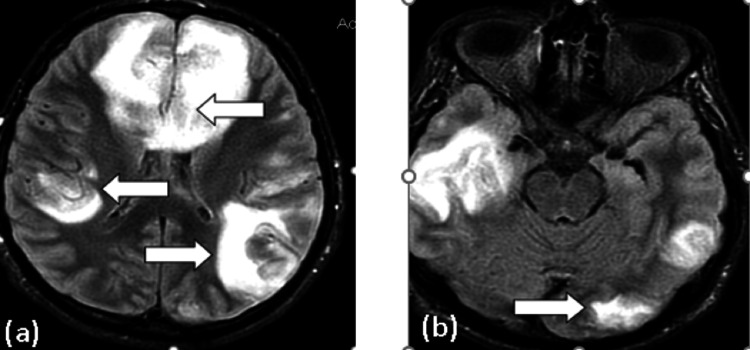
Axial non-enhanced MRI of the brain FLAIR sequences. Bilateral confluent and nodular cortex in the fronto-parieto-temporal (a) and left occipital lobes (b), accompanied by surrounding edema (arrows) MRI: magnetic resonance imaging, FLAIR: fluid-attenuated inversion recovery
